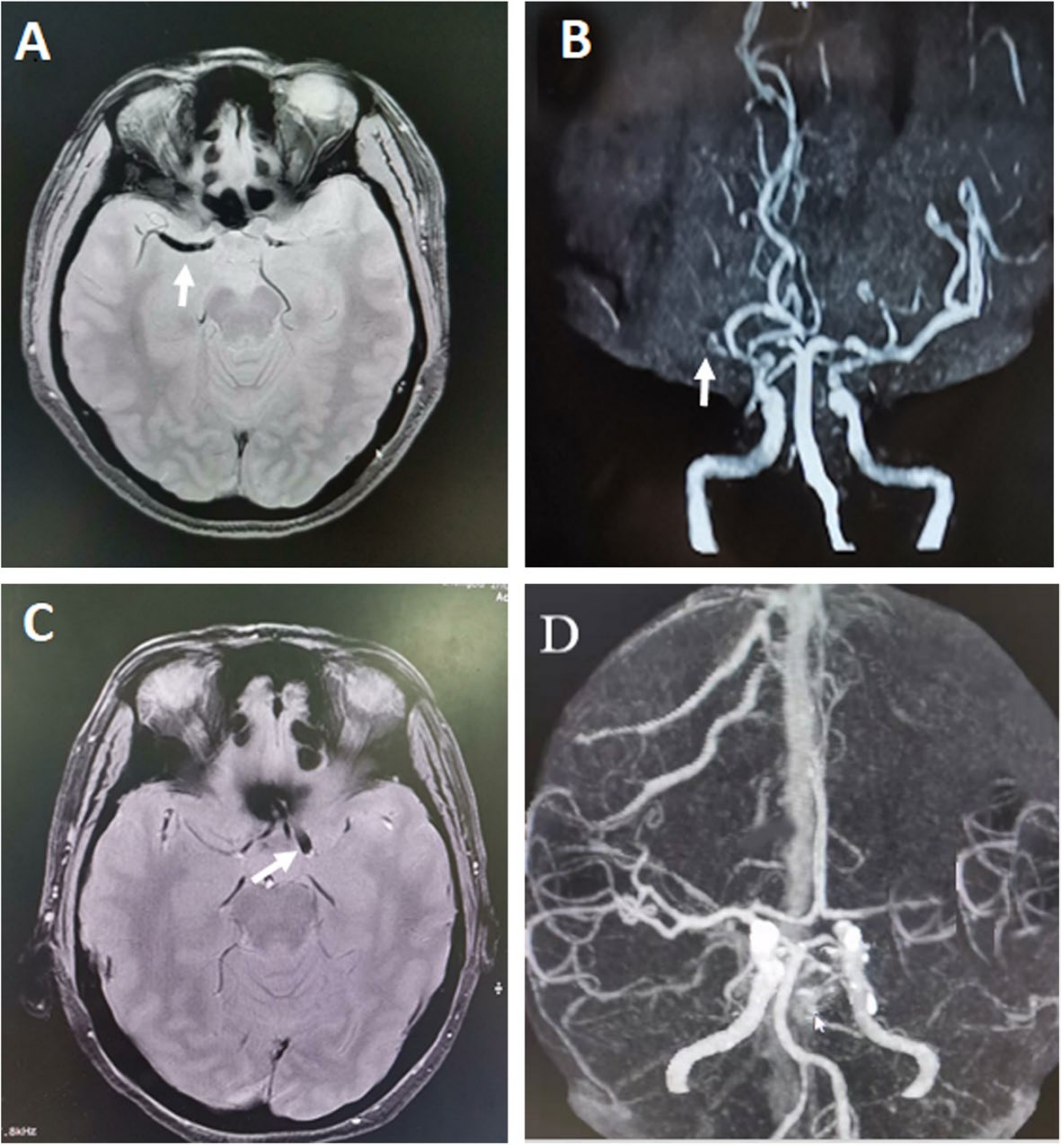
Examples of long SVS and short SVS. MRI of 2 patients presenting with long SVS (A and B, white arrow) and short SVS (C and D, white arrow); A and C, axial views of T2*-GRE; B, MRA; D, CTA
